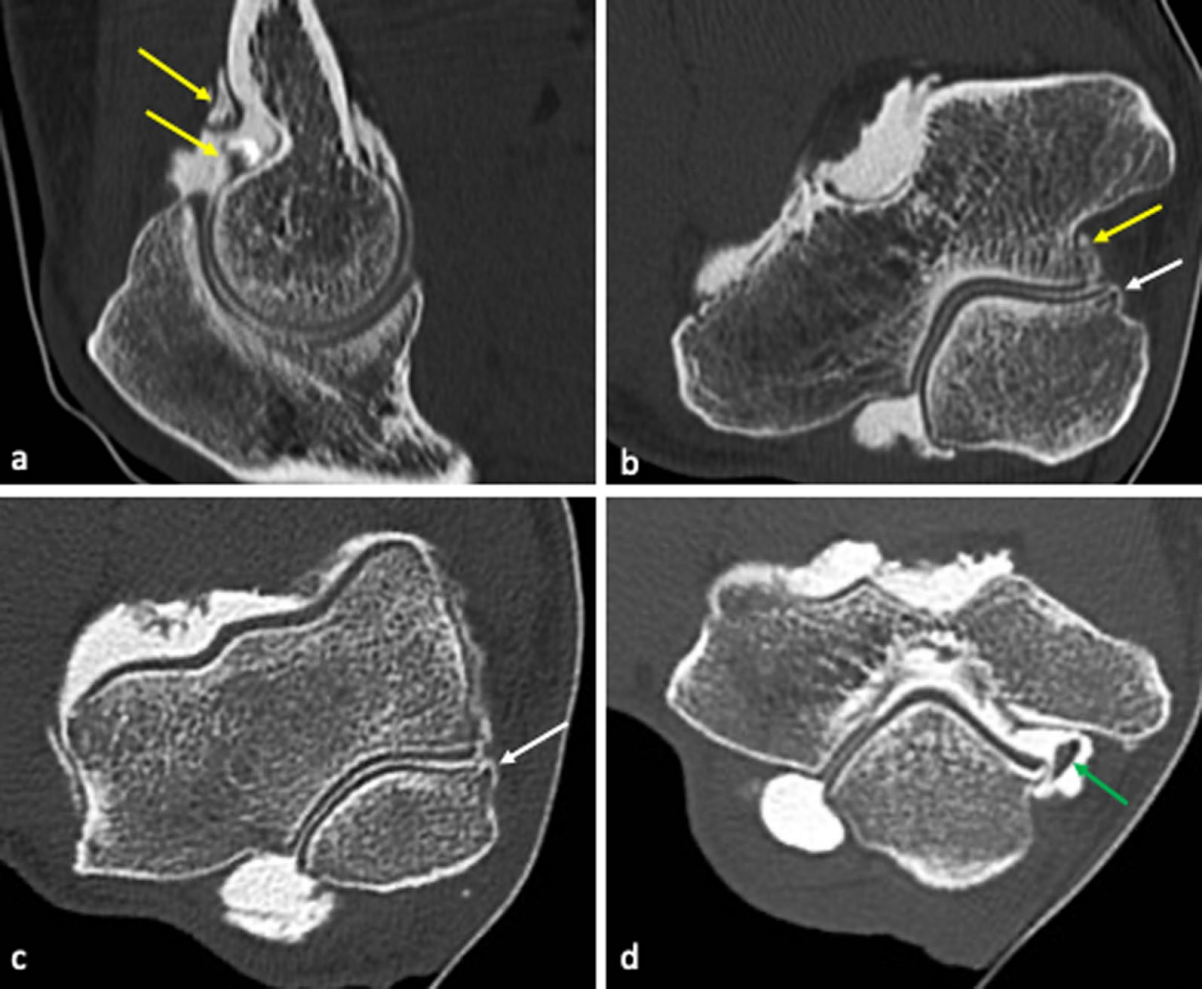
CT-arthrography sagittal (a) and axial (b–d) images of two different patients with valgus extension overload (VEO) syndrome, showing osteophyte formation (b, c) over the medial aspect of the olecranon (white arrows) and an intra-articular loose body (d) located medially at the posteromedial recess (green arrow). Synovial thickening associated to synovitis (a, b) is also noticeable (yellow arrows)
